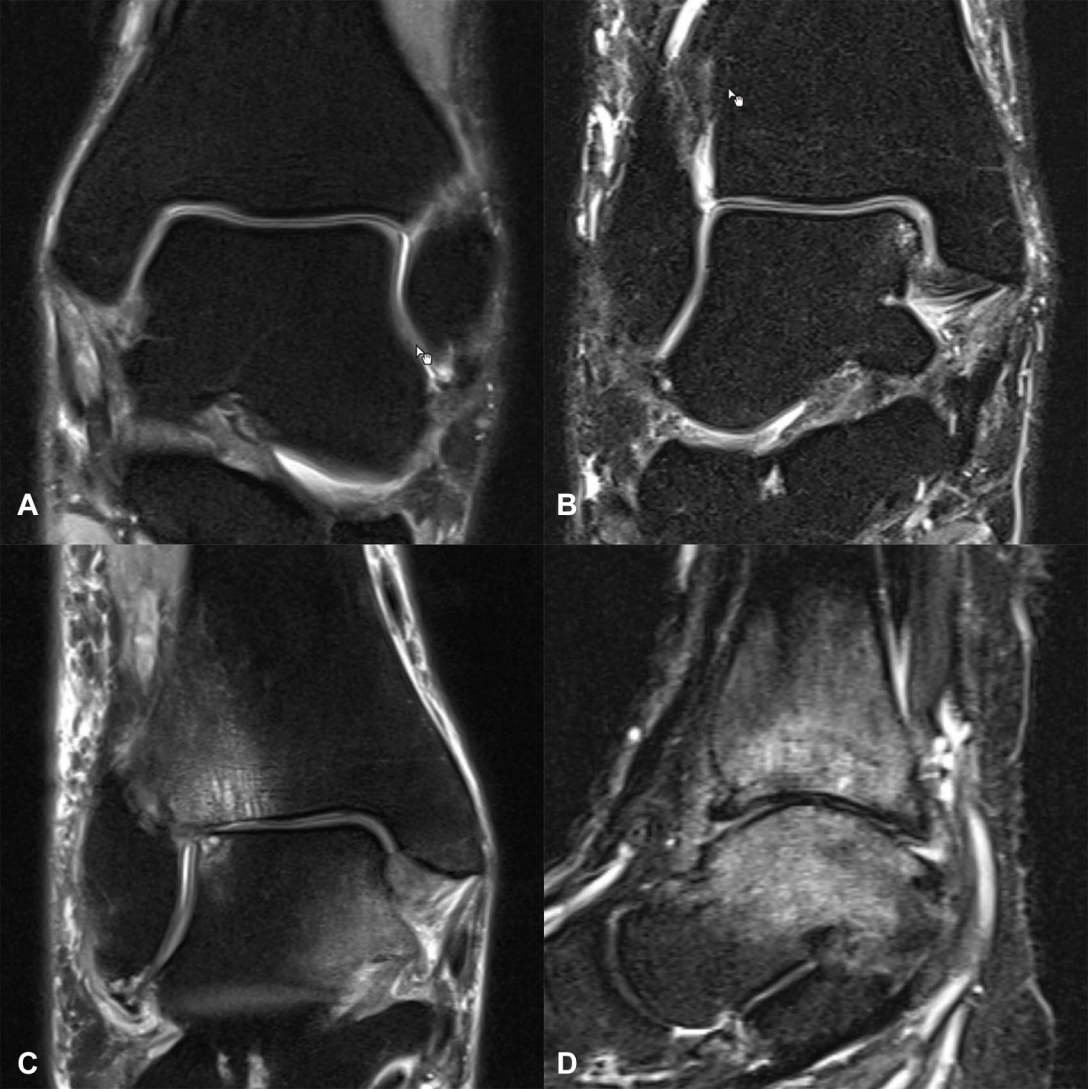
Grading of the presence and extent of BME-like signal on fluid-sensitive magnetic resonance images of 4 different patients: (A) none; (B) mild BME with slight involvement of only 1 articular side, no involvement more than 1 cm below cartilage surface; (C) moderate BME with both joint sides involved, involvement also >1 cm below the cartilage surface; (D) severe BME involving both sides of the cartilage surface. BME, bone marrow edema.
